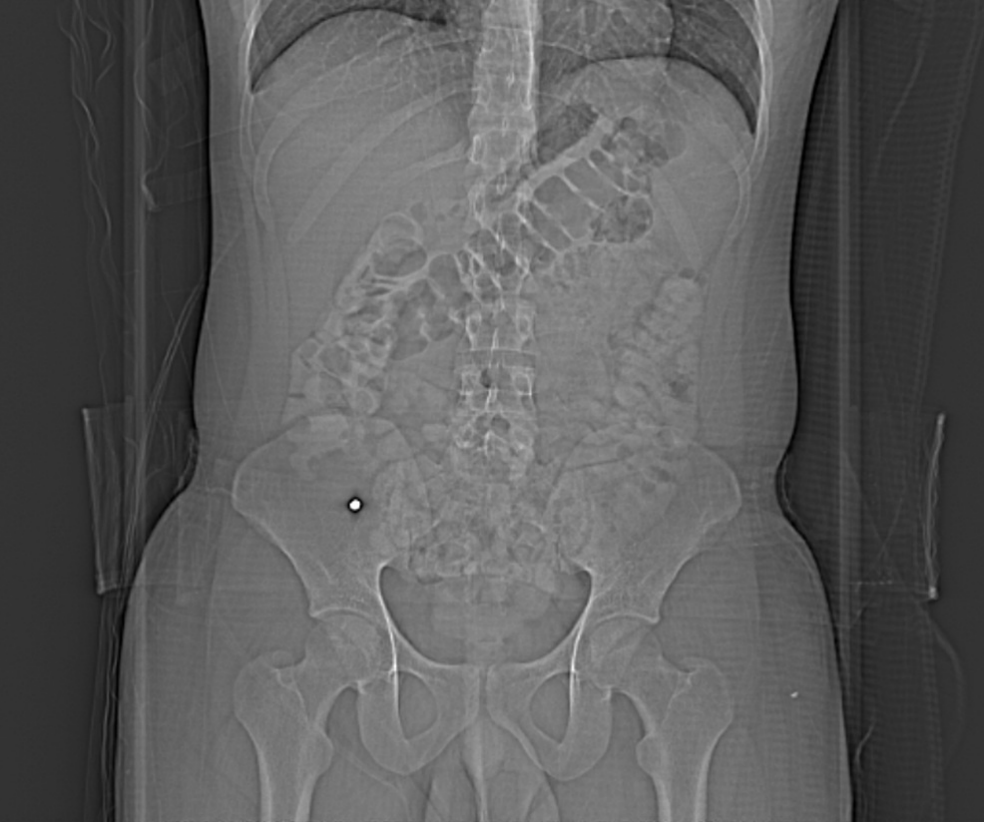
Scout Image The scout image of the CT scan with a round metallic density foreign body in the right lower quadrant
